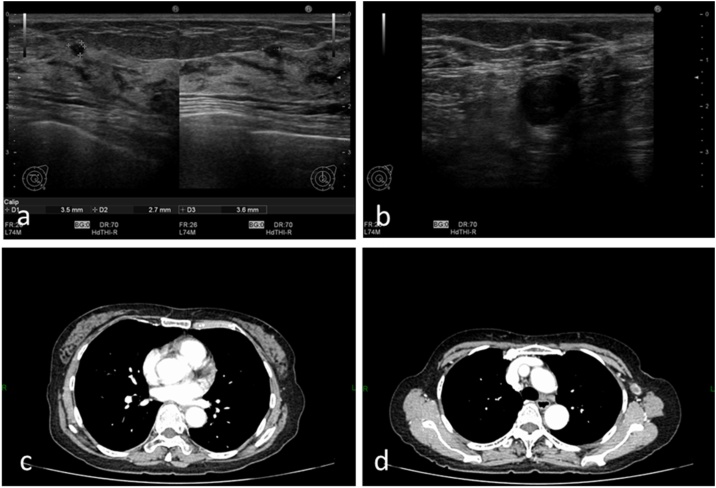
Imaging findings obtained at another university hospital. (a) Ultrasonography revealed a circular tumor in the upper outer region of the left breast, with a clear boundary and internal hypoechoic lesion. (b) Ultrasonography also revealed a circular lymph node with a 15- × 15-mm hypoechoic lesion in the left axilla. (c) Contrast-enhanced computed tomography (CT) revealed point-like signals in the upper outer region of the left breast. (d) Contrast-enhanced CT also revealed swollen lymph nodes with contrast-enhanced findings in the left axilla. The center of the lymph node showed poor contrast-enhanced findings.
